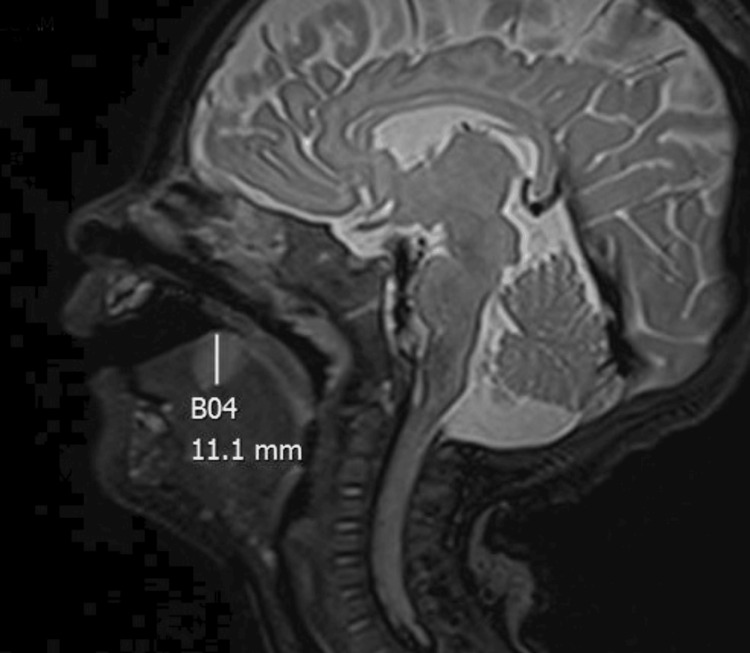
MRI with contrast demonstrating contrast enhancing 1.5 x 1.1 cm mid-tongue mass
